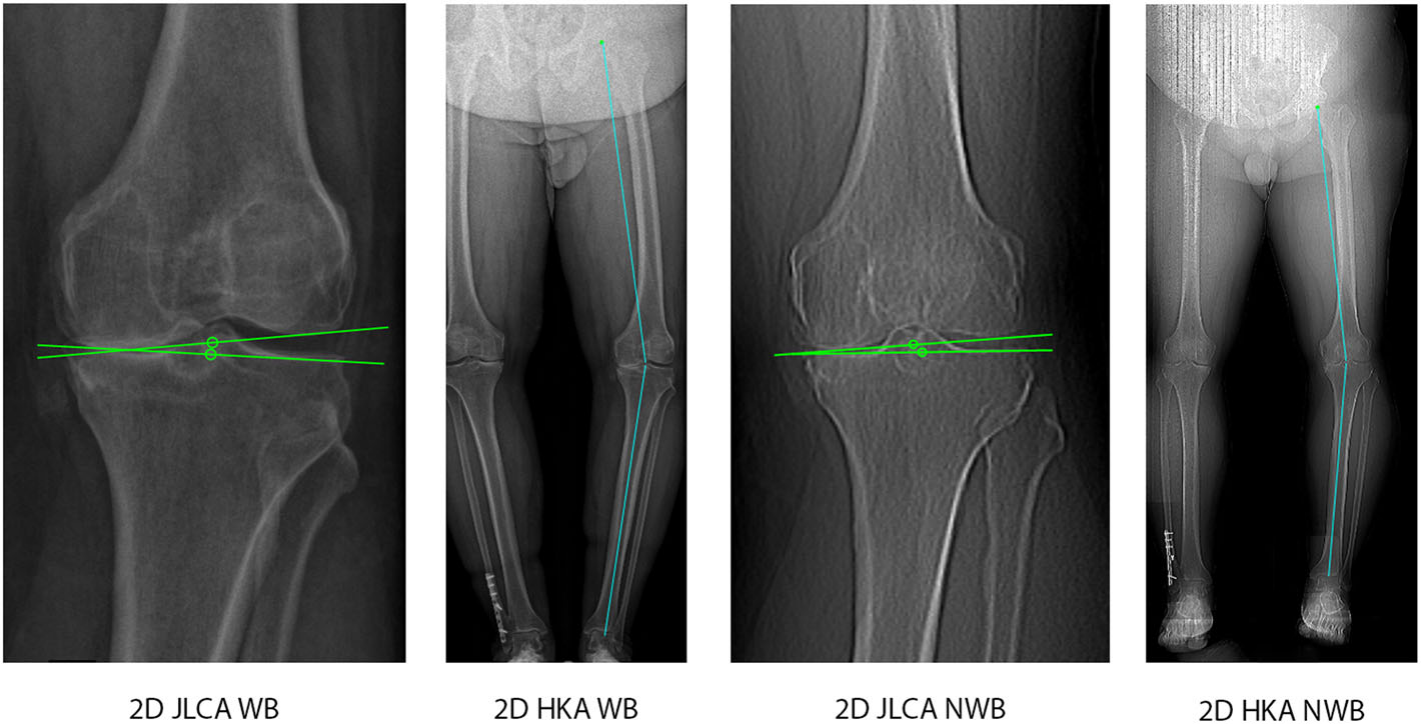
Measurements of 2D HKA and 2D JLCA in WB and NWB conditions. On the left side, measurements were performed using the long-leg radiographs (i.e., WB condition). On the right side, measurements were performed using the scanogram (i.e., NWB condition)
